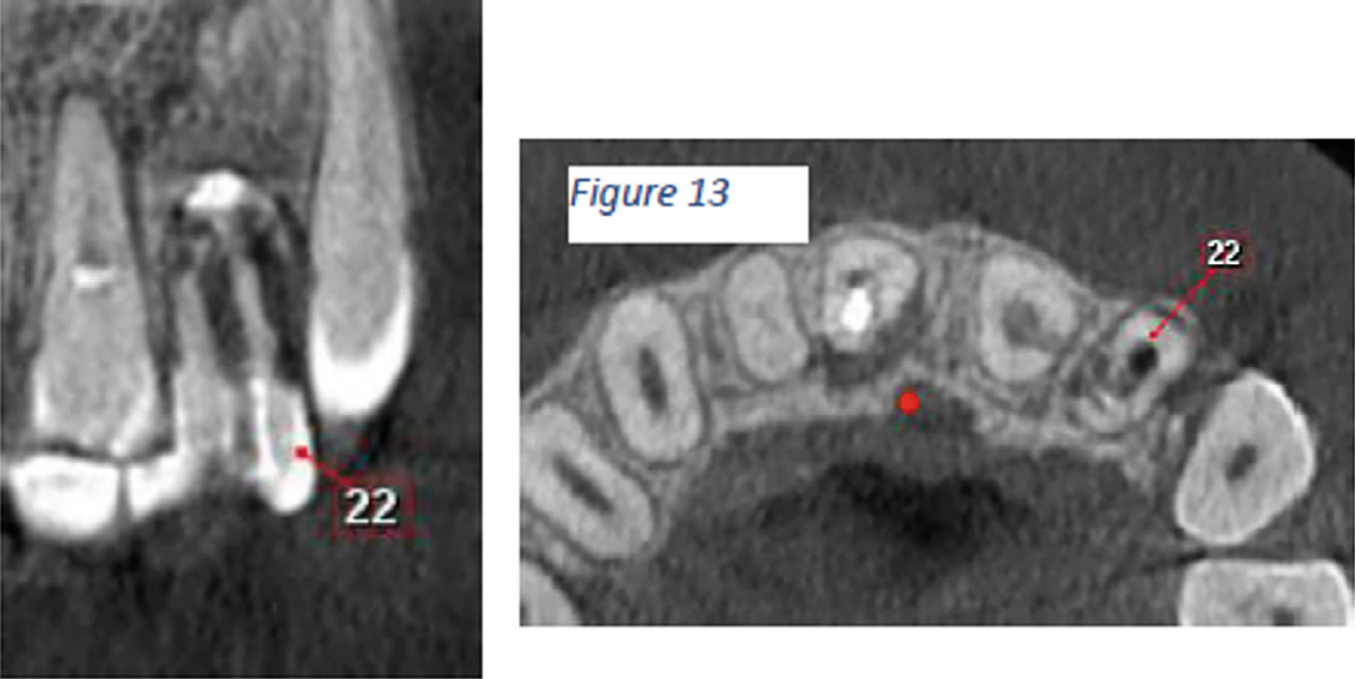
CBCT images showing coronal and axial views of Tooth Number 22 with external root resorption and blunderbuss canal with open apex.
